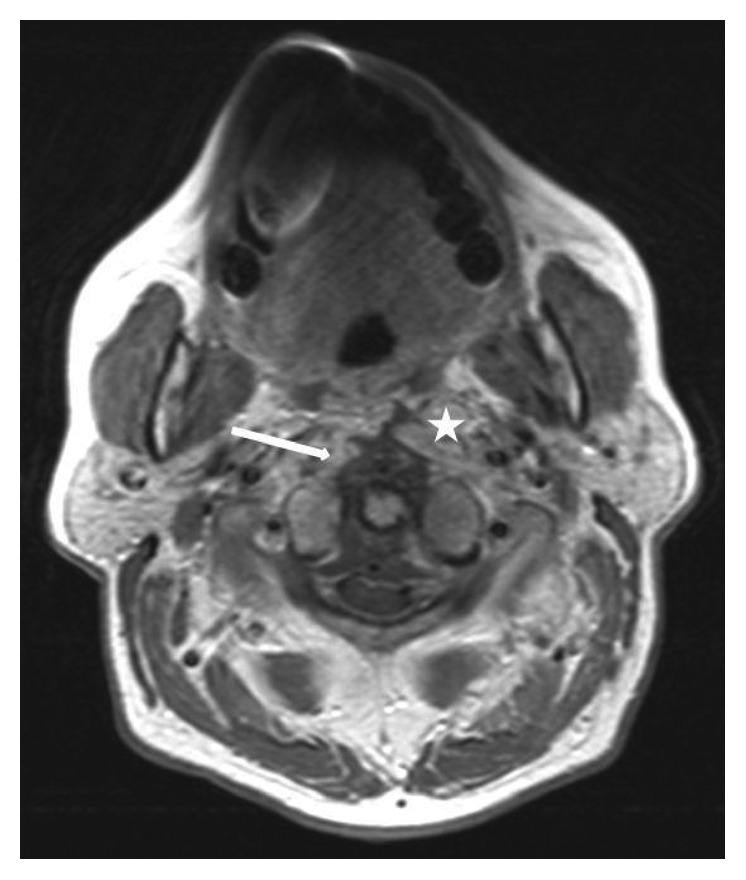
Axial MRI slice five days after incision and drainage of the prevertebral abscess: contrast-enhanced T1-weighted axial MRI scan shows the incised abscess cavity (white asterisk) which is connected to a progressive fluid retention especially in the area between the dorsal ligament and the ventral dura with a slight compression of the myelon (white arrow). This epidural abscess ranged from the foramen magnum to the upper ridge of the third cervical vertebra.
